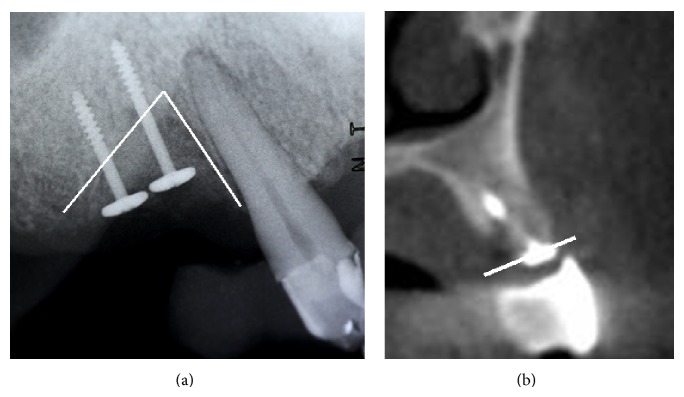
(a) Tenting screw technology for implant # 5 site development. (b) Significant bone increase in vertical and horizontal dimensions, confirmed by the CBCT sagittal slice.
